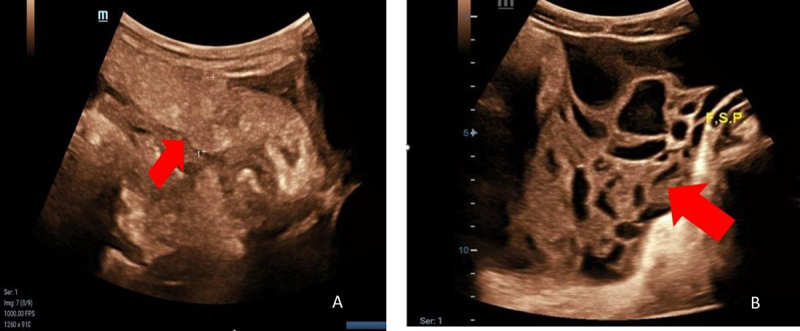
Abdominal ultrasound highlighting acute inflammatory changes
